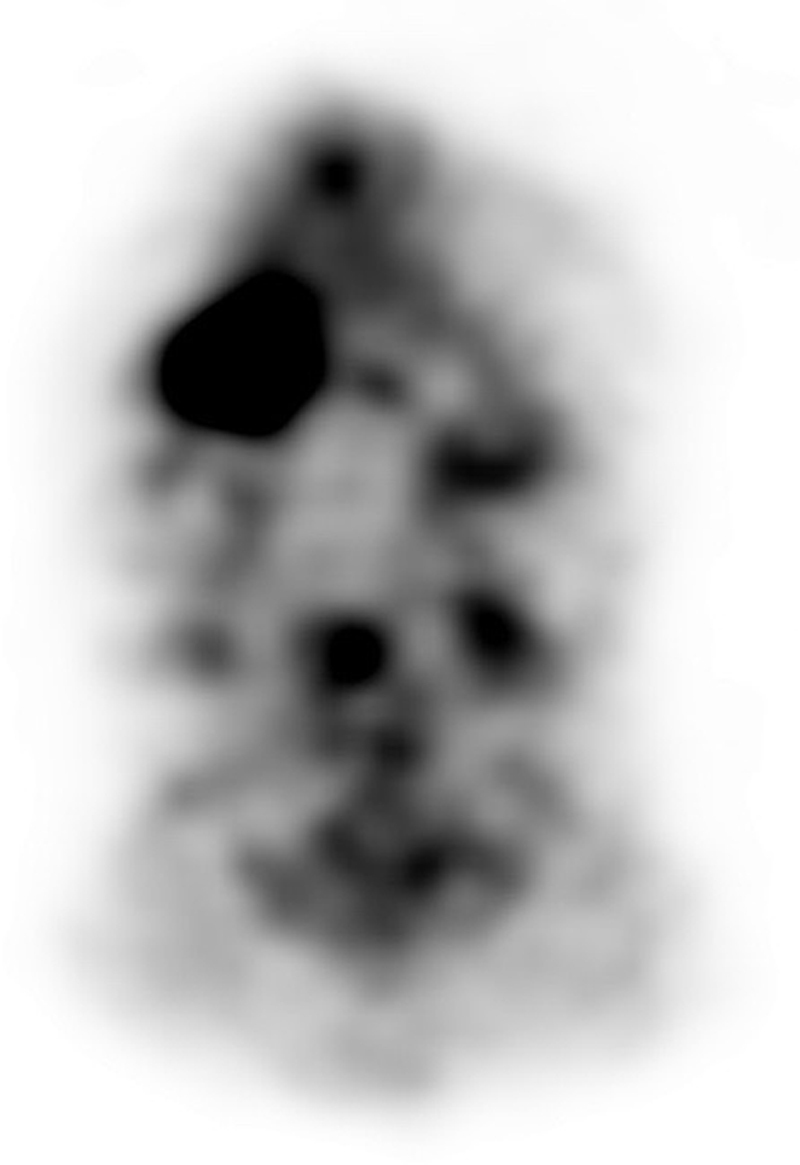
Whole-body magnetic resonance imaging (MRI) with coronal short TI inversion recovery (STIR) sequences (case 2). A, Increased signal intensity of thoracic and lumbar vertebral bodies, spinous processes, and right cotyl. B and C, Radiological control 6 months later: disappearance of these hypersignals.
